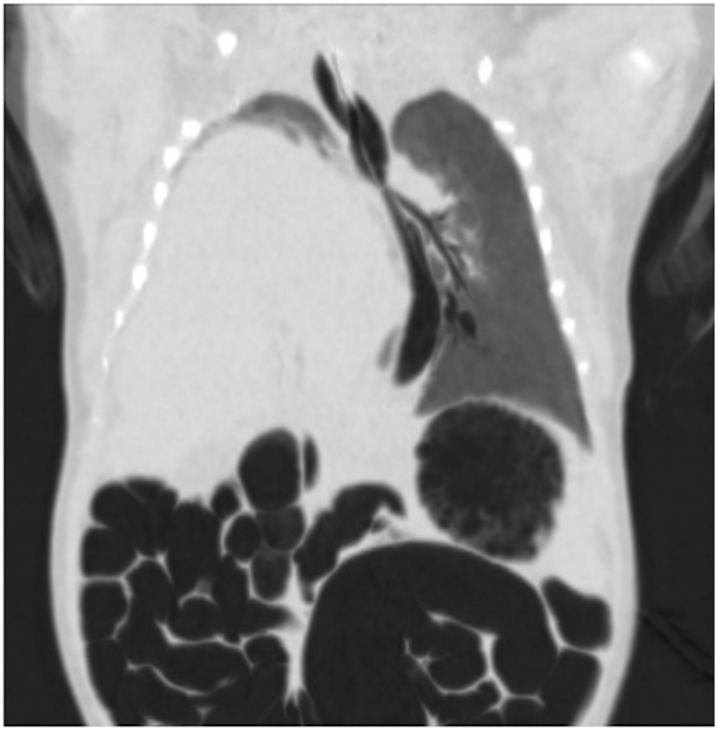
Confirmatory chest CT scan showing a right congenital diaphragmatic hernia with an intrathoracic location of the liver.
